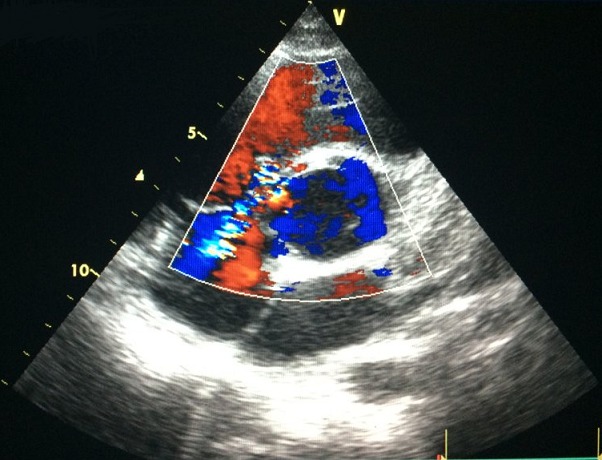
Incidence parasternale petit axe montrant un shunt gauche-droit interventriculaire au doppler couleur
